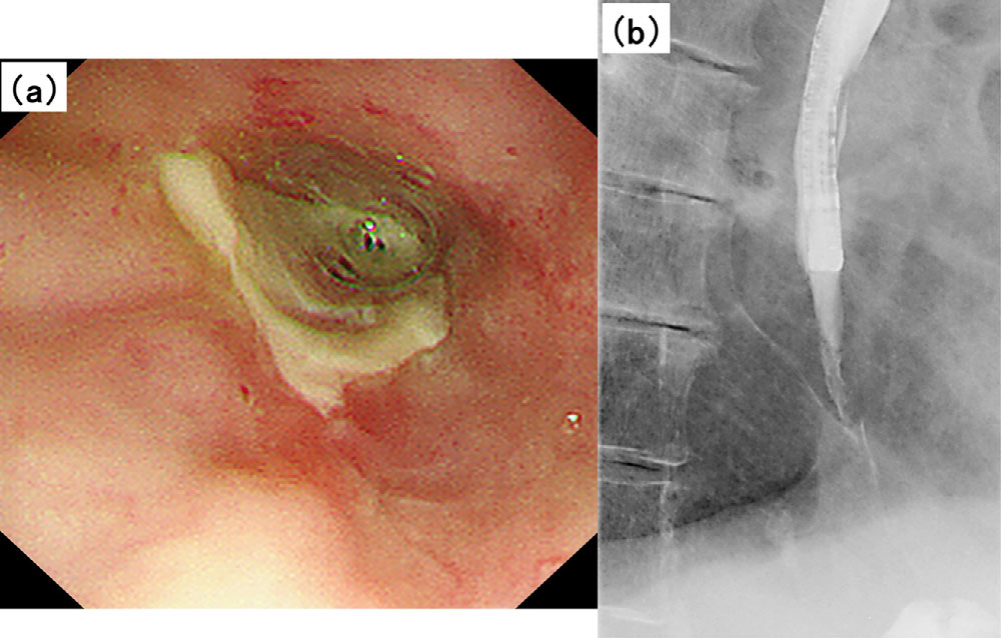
(a, b) At the time of the third admission, EGD and radiography examination showed no improvement in esophageal ulcers and strictures, impeding passage of the endoscope. EGD, esophagogastroduodenoscopy.
